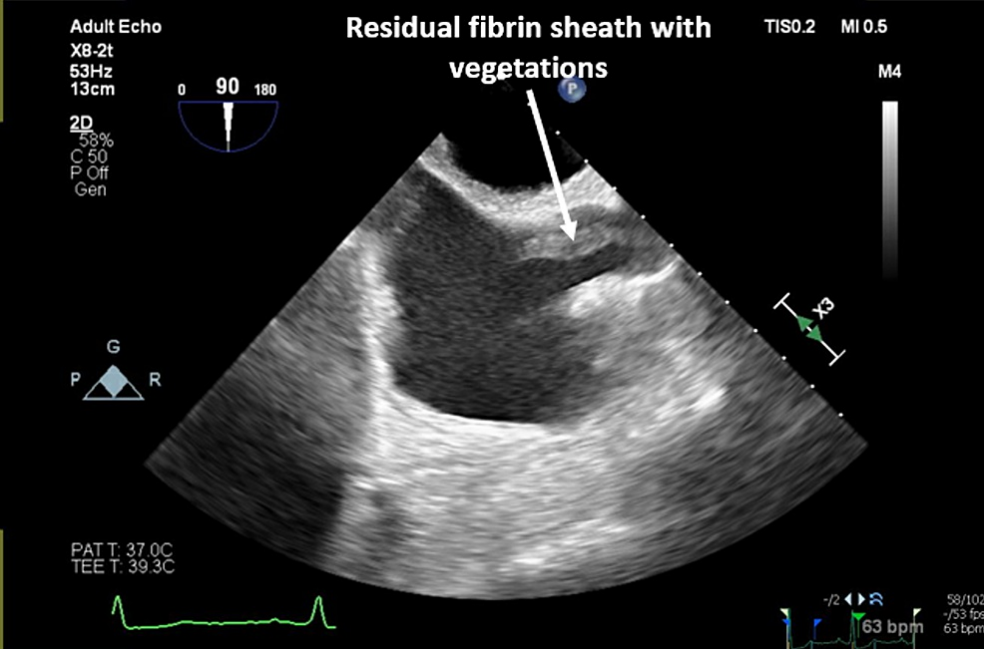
Transesophageal echocardiogram showing fibrin sheath with vegetations (after catheter removal).
